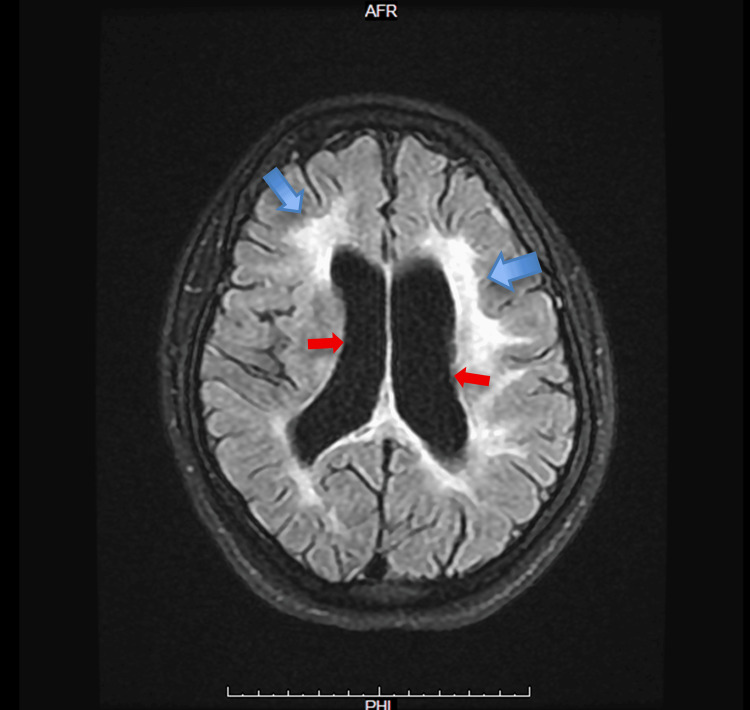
Axial T2 FLAIR MRI of the brain with contrast The axial T2 FLAIR sequence post-contrast demonstrates diffuse periventricular and deep white matter hyperintensities surrounding the lateral ventricles (blue arrows). The ventricles are enlarged relative to patient age (red arrows), consistent with reported cortical atrophy. No abnormal contrast enhancement is observed, suggesting the lesions are non-acute and non-enhancing. Findings are compatible with a demyelinating or inflammatory white matter process, as described in the clinical report MRI: magnetic resonance imaging; FLAIR: fluid attenuated inversion recovery
